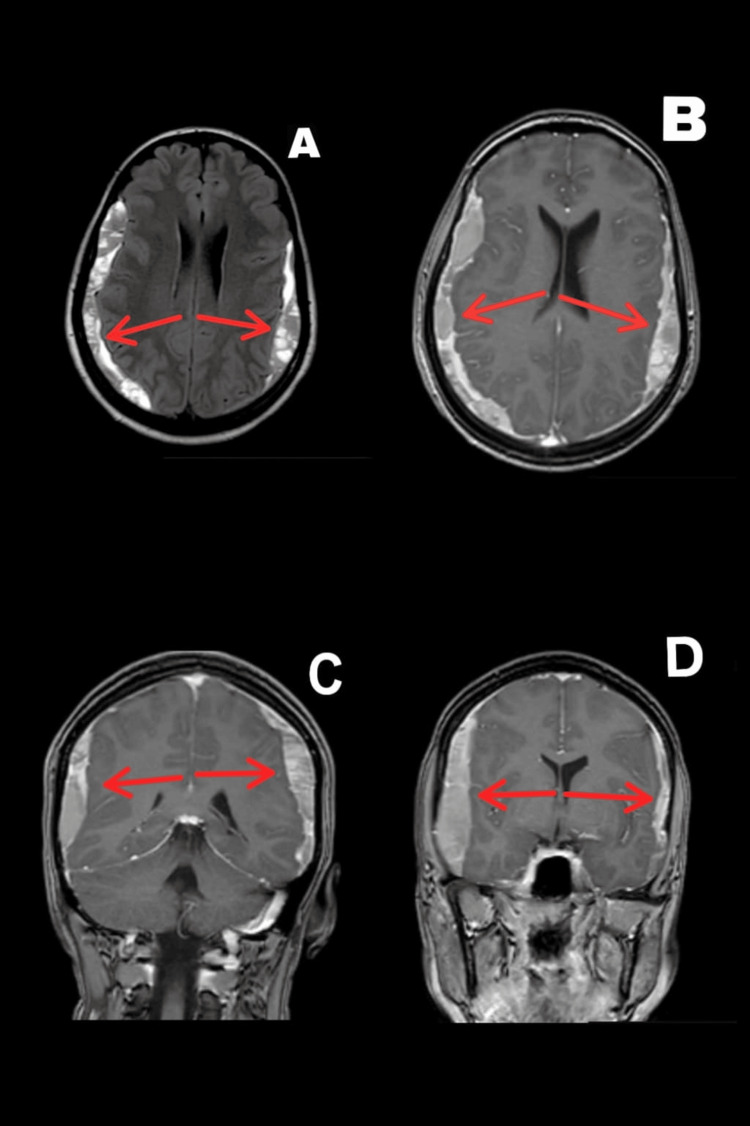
Brain MRI showing bilateral subdural hemorrhage of different ages Figures 1A-1B show the axial view. Figures 1C-1D show the coronal view MRI: magnetic resonance imaging
